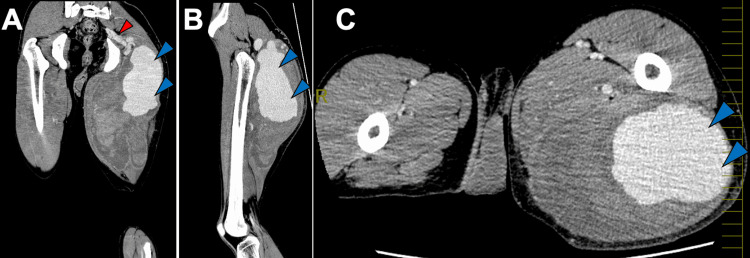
Contrast-enhanced CT obtained on arrival at the emergency room: (A) coronal, (B) sagittal, and (C) axial views. Extensive contrast extravasation (panels A-C, blue arrows) within the posterior proximal thigh hematoma, indicating acute bleeding, is observed. Marked dilation of the internal iliac artery and inferior gluteal artery (panel A, red arrow), appearing continuous with the lesion, is also noted.
